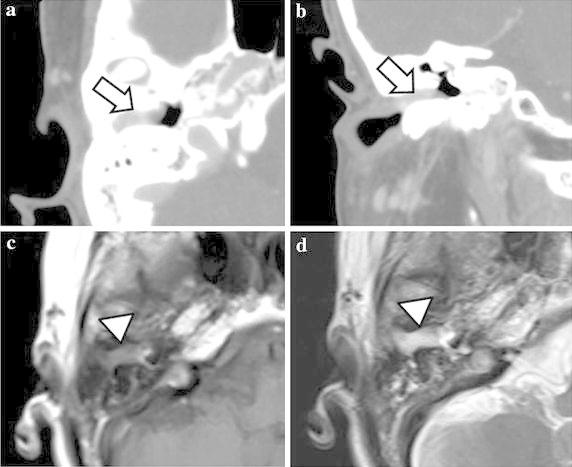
Case 1: Enhanced CT scan of temporal bone, axial view a and coronal view b demonstrates a soft-tissue mass (*arrow*) lateral to the tympanic membrane that fills the right external auditory canal without involvement of the tympanum. There is no evidence of bone erosion, and the tympanic membrane, middle ear, and ossicles appear normal. Magnetic resonance imaging (MRI) of the skull base shows an external auditory canal mass (*arrowhead*) that is slightly hypointense in a T1-weighted image (c), and hyperintense in T2-weighted images (d).
